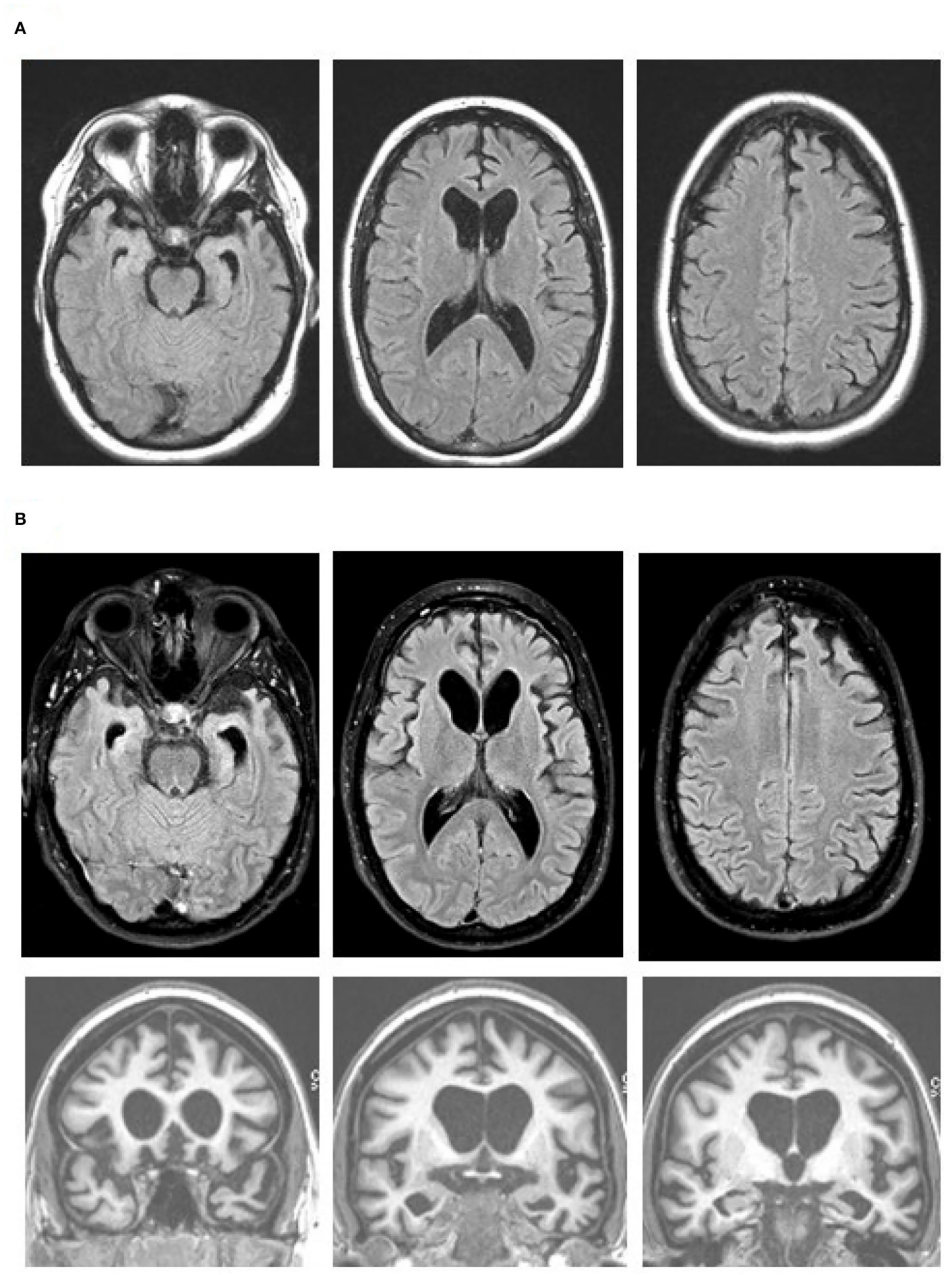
Brain MR images at age 27 (A) and 19 months later (B), with axial fluid attenuation inversion recovery (FLAIR) images (top and middle rows) and coronal T1-weighted images (bottom row). Note progression of the bilateral medial frontal, anterior temporal, and caudate head atrophy with the associated ventricular enlargement on the axial FLAIR images. The orbitofrontal as well as dorsomedial, anterior temporal, and caudate head atrophy are obvious on the coronal images. Images are in standard radiology orientation.
